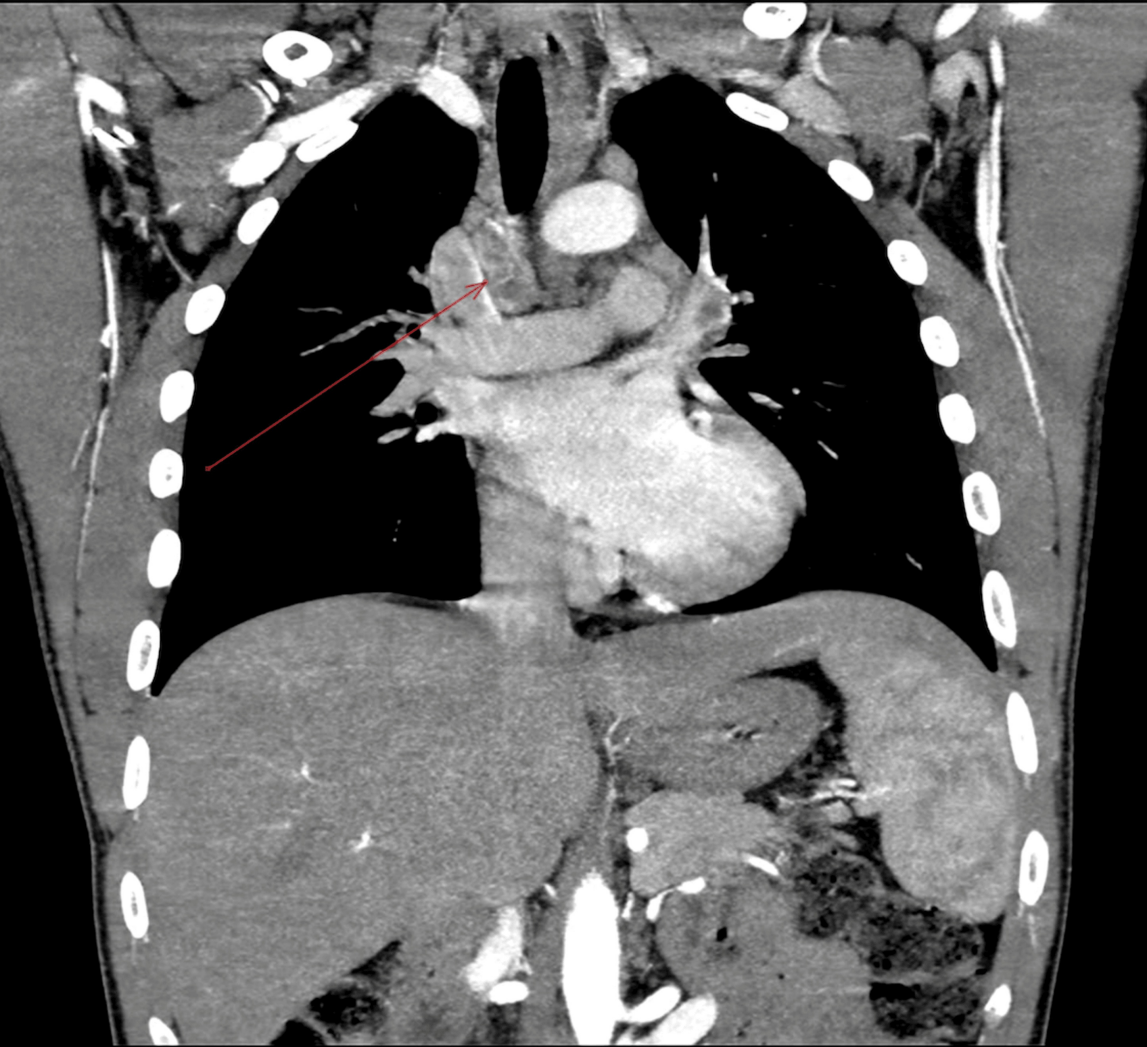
CT pulmonary angiography showing multiple necrotic mediastinal lymph nodes
